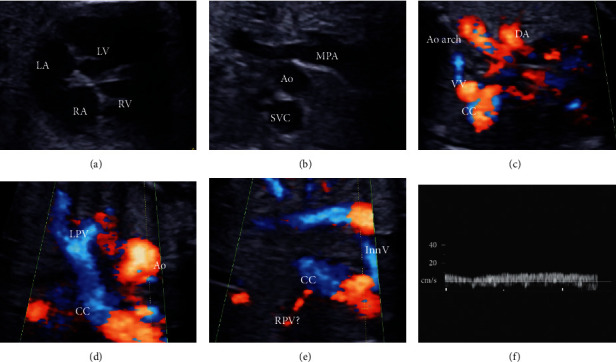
Foetal echocardiography. (a) An atrial septal defect is detected. (b) The superior vena cava is enlarged. (c) Vertical vein (VV) flow from the common chamber (CC) shows a continuous pattern. (d) Left pulmonary vein (LPV) flows into the enlarged CC. (e) The right PV (RPV) seems to flow to the CC. (f) The RPV shows a continuous flow pattern. The A-dip of the flow is seen to disappear. Ao, ascending aorta; Ao arch, aortic arch; DA, ductus arteriosus; InnV, innominate vein; LA, left atrium; LV, left ventricle; MPA, main pulmonary artery; RA, right atrium; RV, right ventricle.
